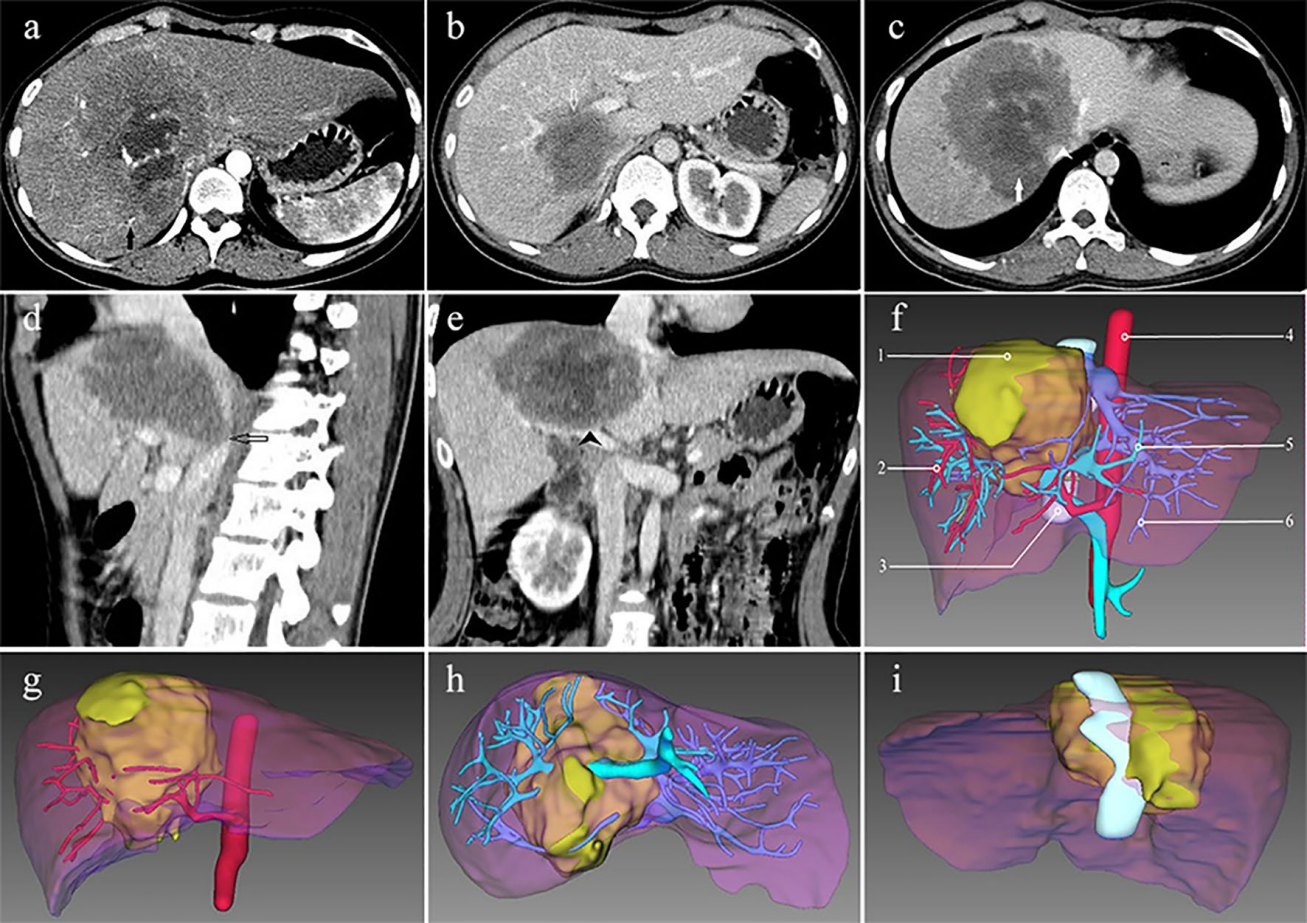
CT of a large hepatic alveolar echinococcosis located in the right liver lobe, caudate lobe and left medial lobe in case 21. Axial CT of the arterial phase (a) demonstrates that the right hepatic artery is of mild invasion by the lesion. Axial CT of the portal venous phase (b) displays the invasion of right portal vein with severe involvement (hollow arrow). Axial CT of the delayed phase (c) reveals obliteration of the right hepatic veins (arrow), and invasion of the second porta hepatis (arrowhead). Sagittal reconstruction of the portal venous phase data (d) displays severe invasion of retrohepatic vena cava (hollow arrow). Coronal reconstruction of the portal venous phase data (e) indicates the invasion of the first porta hepatis (arrowhead). Three-dimensional reconstruction image derived from the portal venous phase data (f) shows the location of the lesion and the relationship between the lesion and hepatic artery, portal vein, hepatic vein and inferior vena cava. The three-dimensional reconstruction images from arterial phase (g) and from the portal venous phase (h and i) data reflect the relationship between the lesion and hepatic artery, portal vein, hepatic vein and inferior vena cava in multiple-angle views, respectively. *Notes* 1, lesion; 2, hepatic artery; 3, inferior vena cava; 4, abdominal aorta; 5, portal vein; and 6, hepatic vein.
